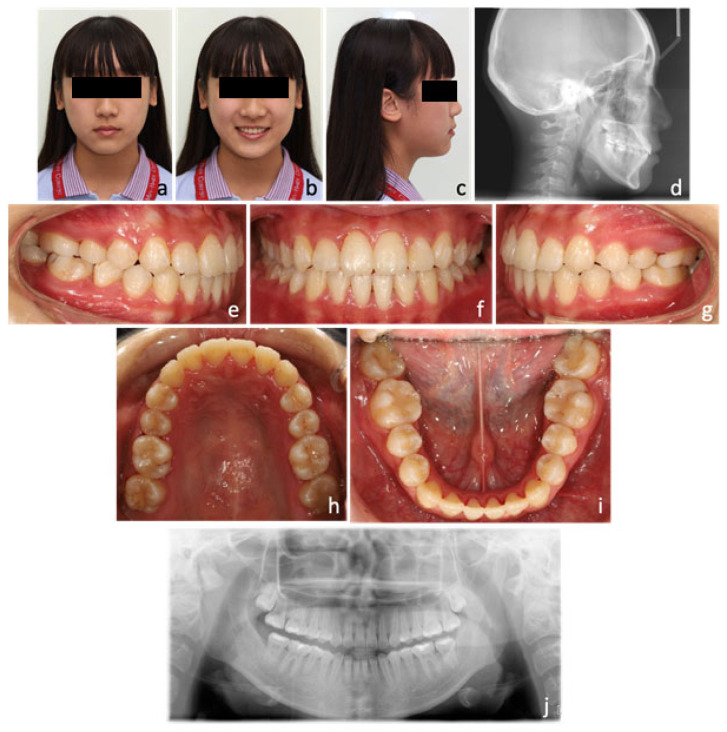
Final photos and radiographs. (a,b) Extra-oral photos showed more upper incisor display and ideal smile arc. (c,d) A straight profile was achieved with less retrusive upper lip to the E-line. (e–i) Intra-oral photos showed the upper narrow arch was expanded and the dental crowding was relieved, anterior and posterior crossbites were corrected and centerlines were coincident. (j) The final orthopantographic (OPG) radiograph showed good root parallelism.
